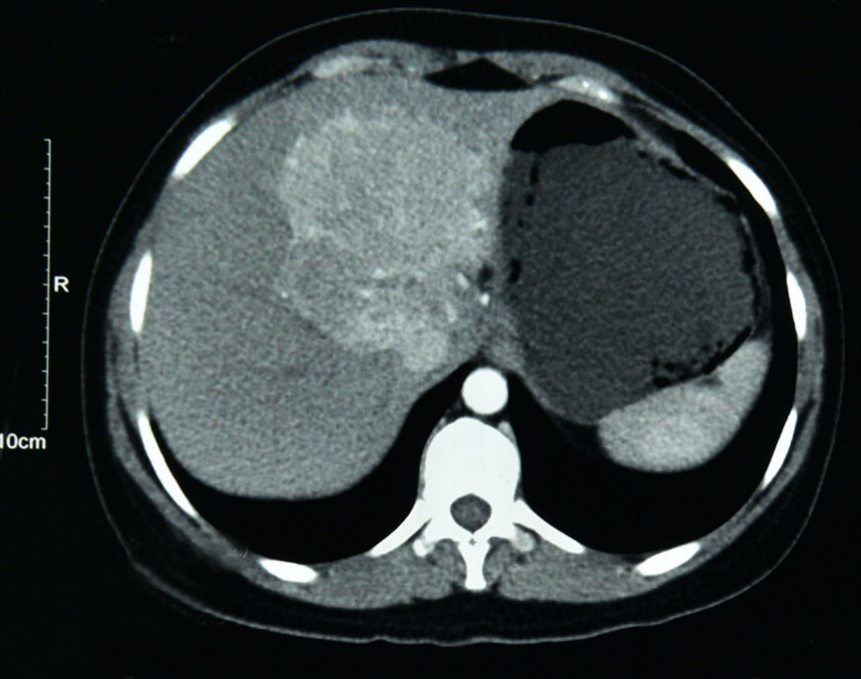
Abdominal computed tomography scan showing a large, lobulated tumor involving the left lobe and part of the right lobe of the liver.
